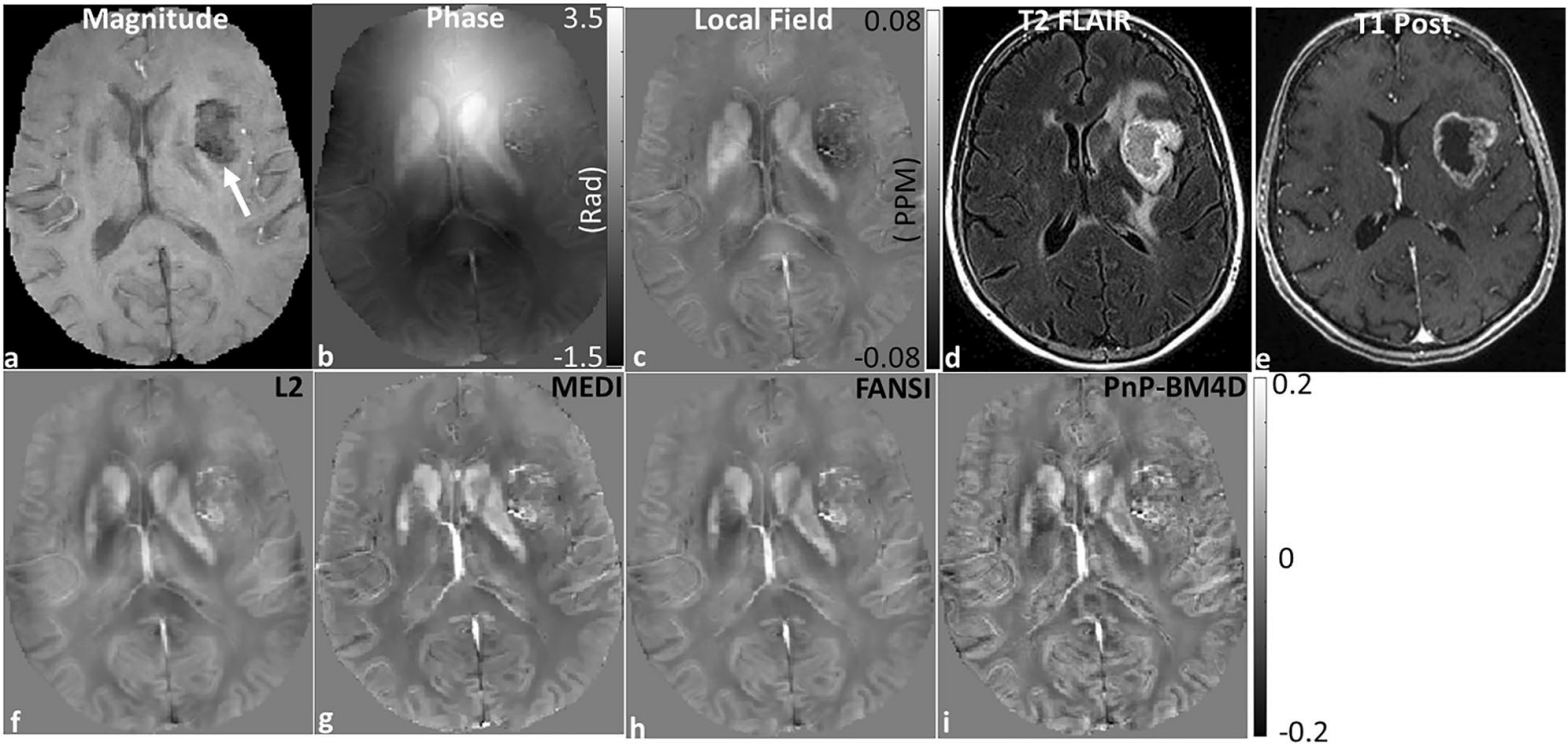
Reconstruction of susceptibility maps from a patient with GBM. (a) The glioblastoma appears hypointense in the T2*-weighted magnitude image (white arrow). The corresponding (b) phase image, (c) local field map, (d) T2 FLAIR and (e) T1 Post contrast image are displayed. QSM reconstructions from (f) closed form L2, (g) MEDI, (h) FANSI, and (i) PnP-BM4D are shown. Tumor features are more conspicuous and show less blurring of vascularity in the PnP-BM4D reconstructions. Additionally, there is less blurring and higher contrast in grey matter cortical regions.
